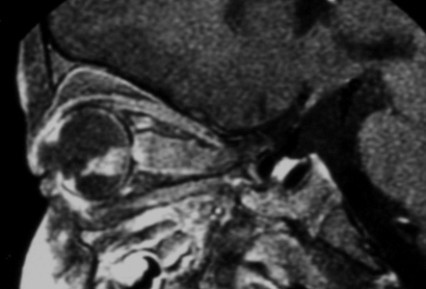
MRI pattern of retinoblastoma with optic nerve involvement (sagittal enhanced T1-weighted sequence).
